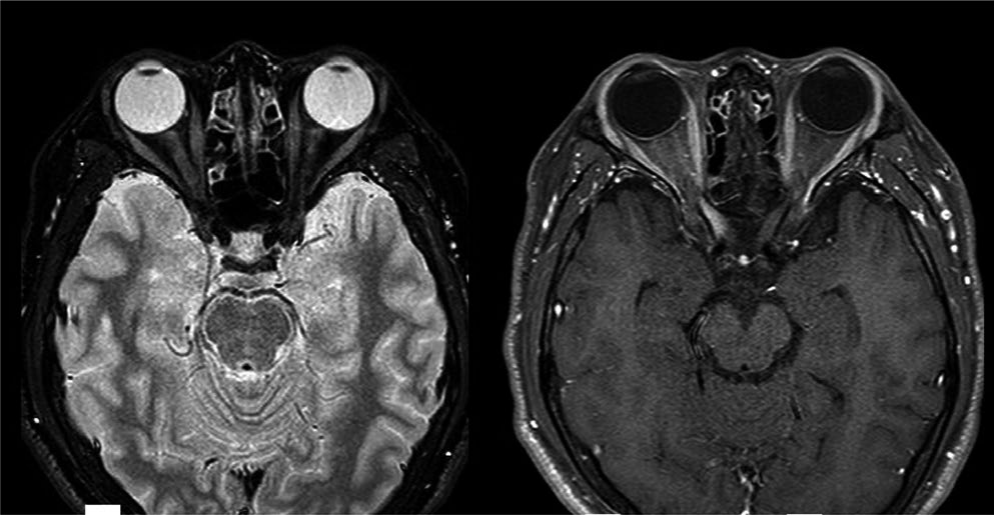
Optic MRI on Day 14 after trauma in Case 1. Optic MRI in the STIR sequence (left) and contrast‐enhanced fat‐suppressed T1‐weighted (right) are shown
